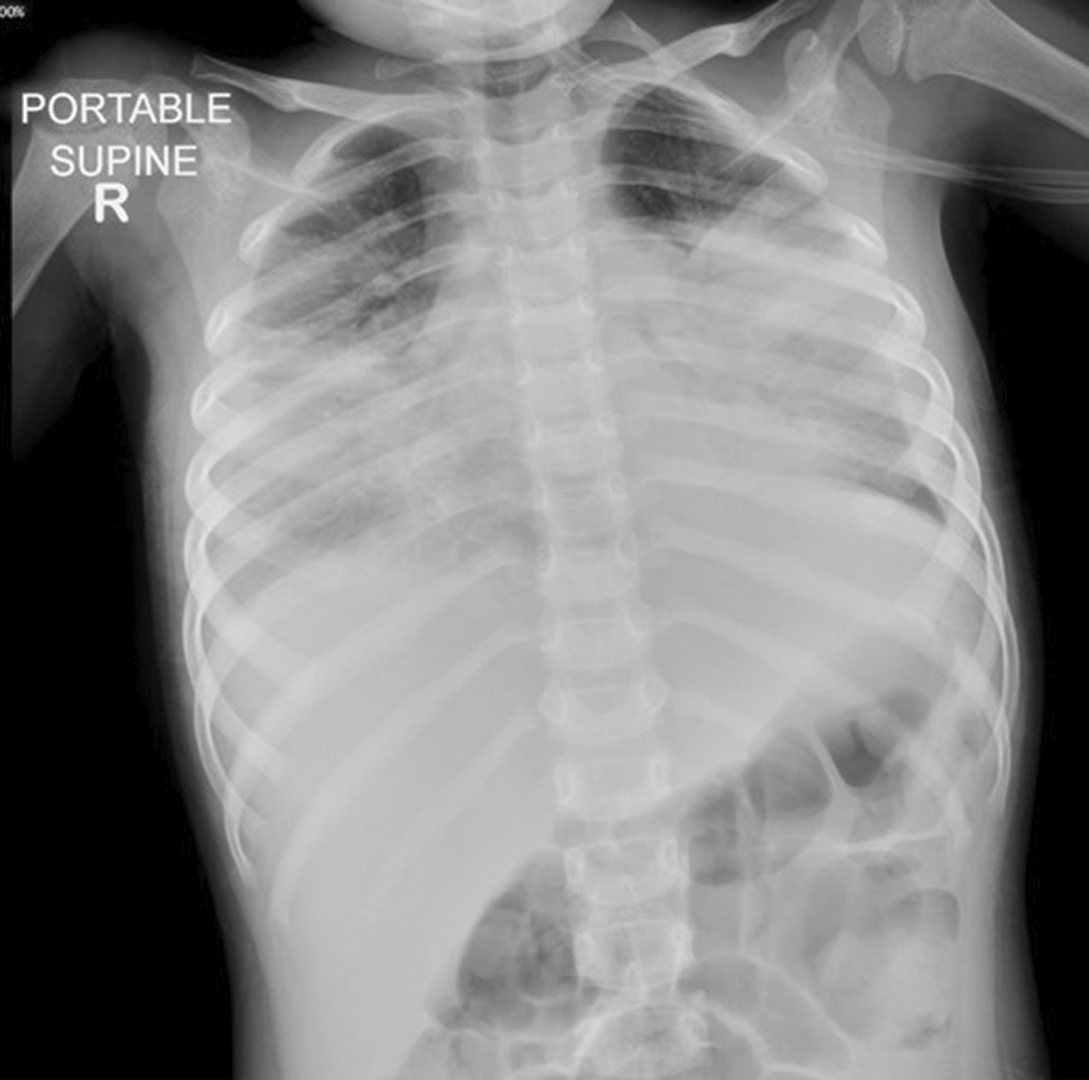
Chest radiograph at initial presentation showing bilateral consolidation and pleural effusion
